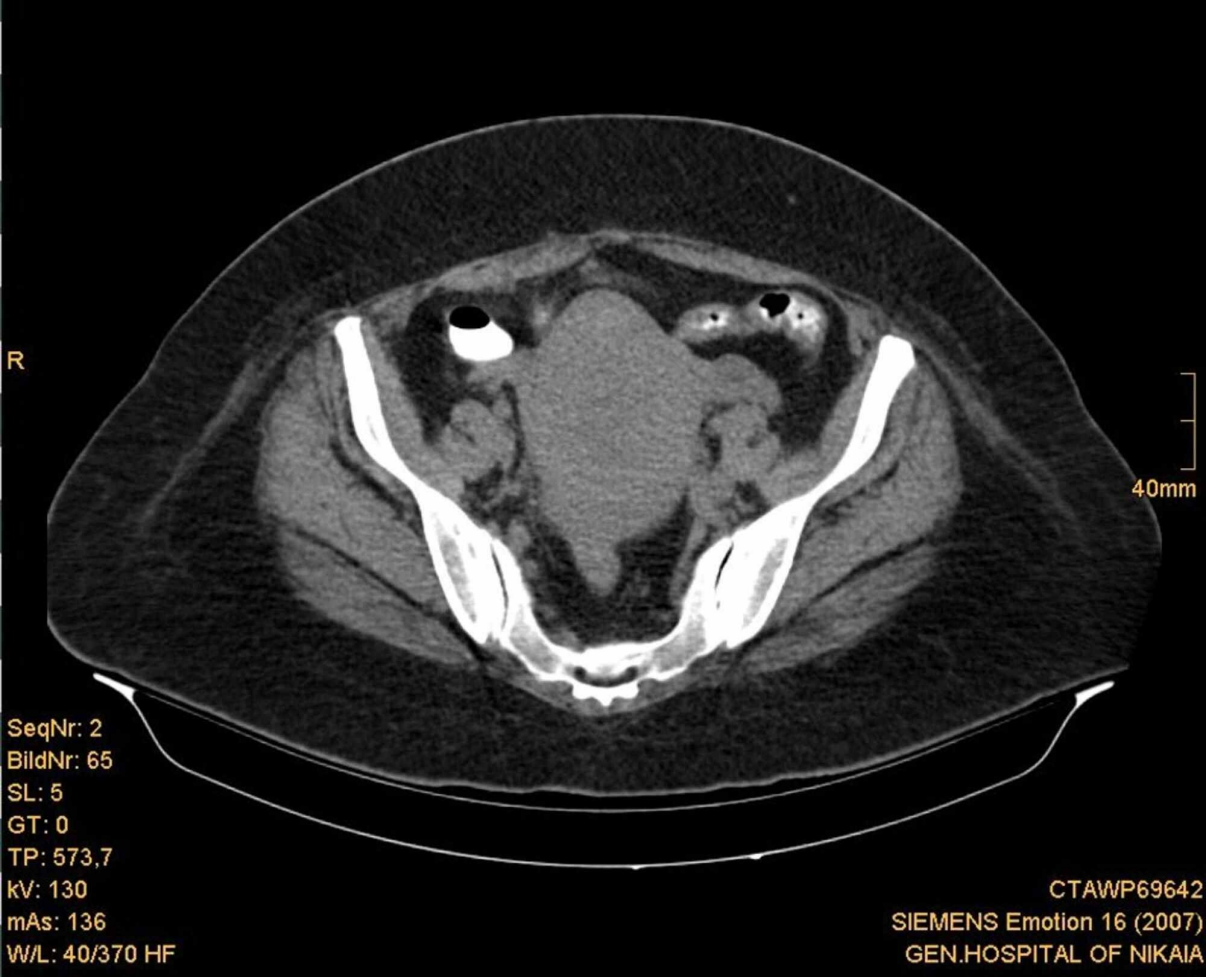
Abdominal CT scan showing the anatomic region of the uterine body.
